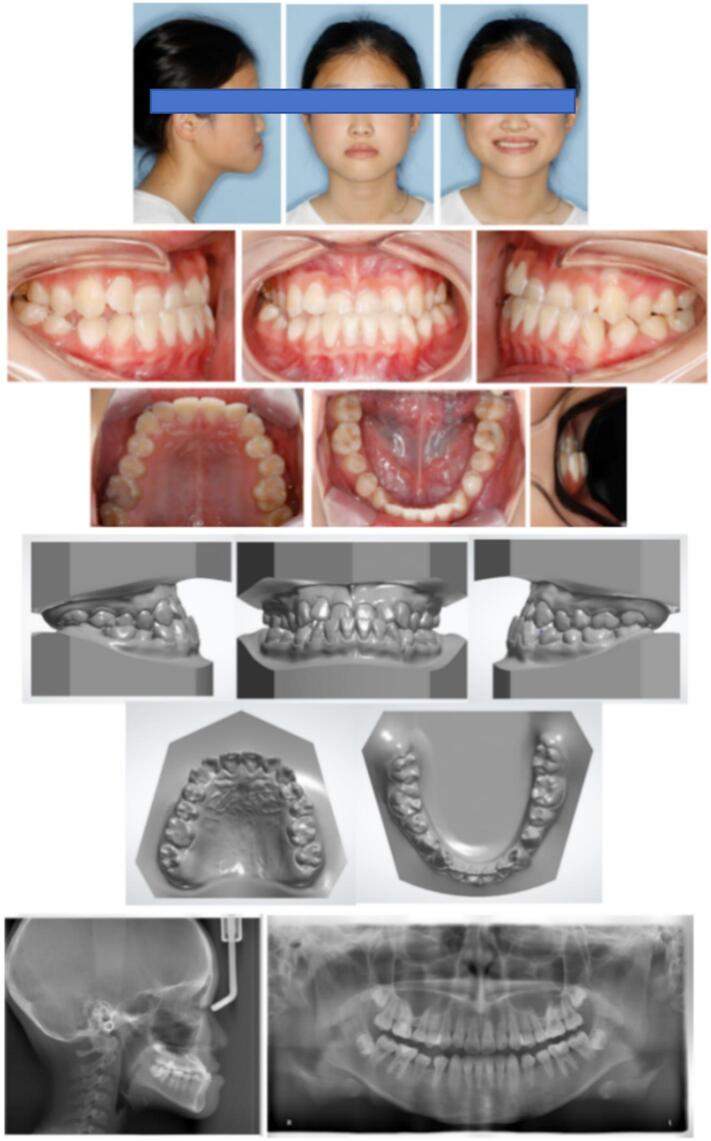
Pre-treatment facial and intraoral photographs, pre-treatment dental casts and pre-treatment radiographs.
